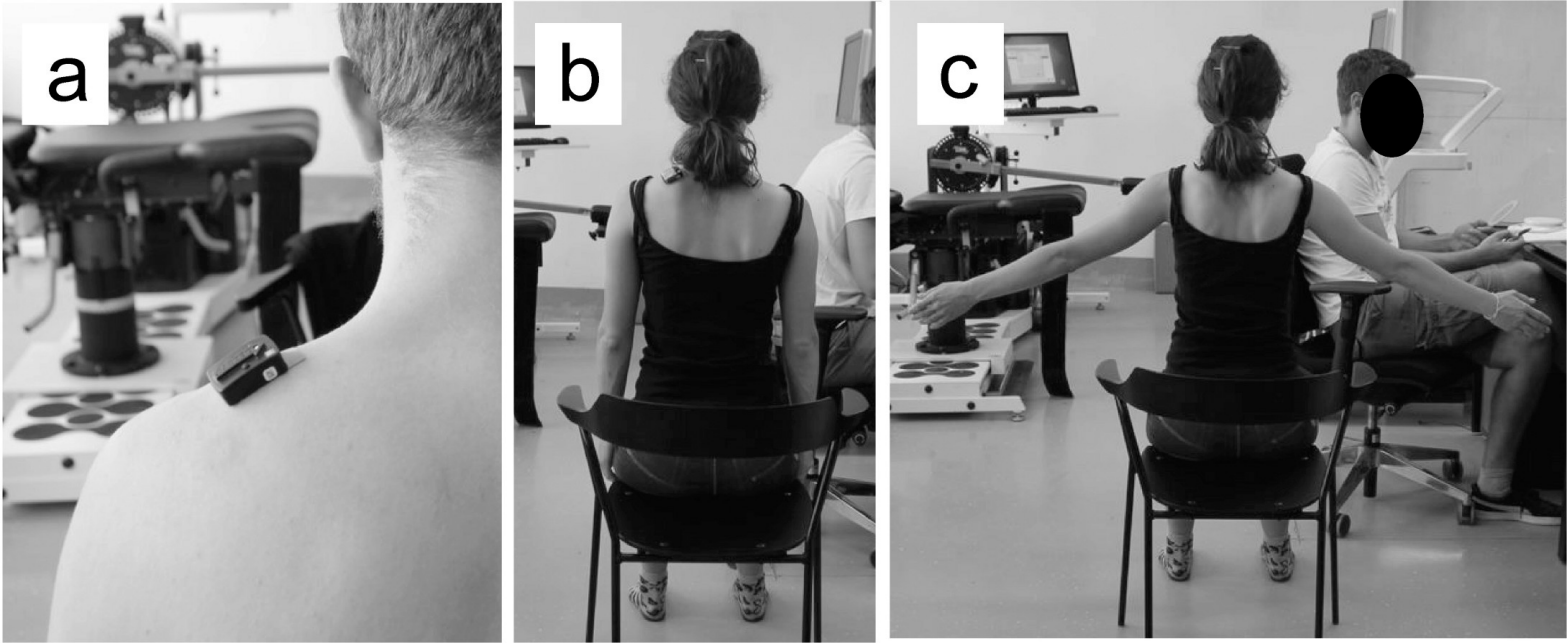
Muscle activity and shear modulus were recorded in the same conditions, with the location of the electrode and the ultrasound probe at 50% of the line from the acromion to the spine of C7 (A). Measurements were performed in a relaxed position (B) and with shoulder abduction of 40° and 60° (C).
